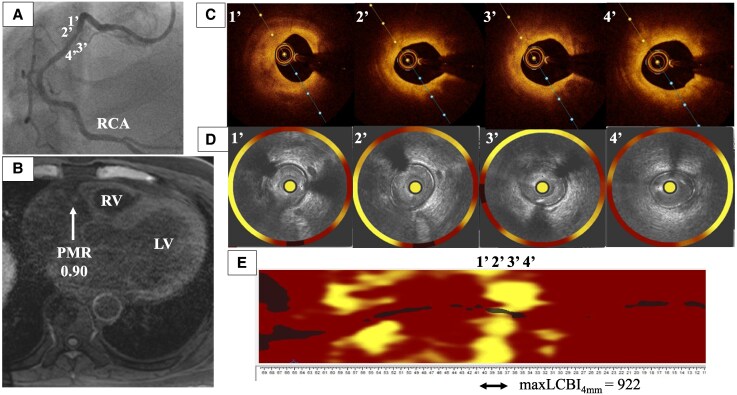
A representative case with target lesion exhibiting PMR < 1.0. A 70-year-old gentleman was hospitalized due to angina pectoris. (*A*) There was a significant stenosis at the middle segment of his RCA. (*B*) PMR at this lesion was 0.90 (white arrow). (*C*) On OCT imaging, the corresponding lesion exhibited lipidic plaque without cholesterol crystal and LIA. (*D* and *E*) PAV on IVUS imaging was 52.4%, and maxLCBI_4mm_ at the corresponding lesion was 922. CMR, cardiac magnetic resonance; IVUS, intravascular ultrasound, maxLCBI_4mm_; OCT, optical coherence tomography; PAV, percent atheroma volume; PMR, plaque-to-myocardium ratio.
